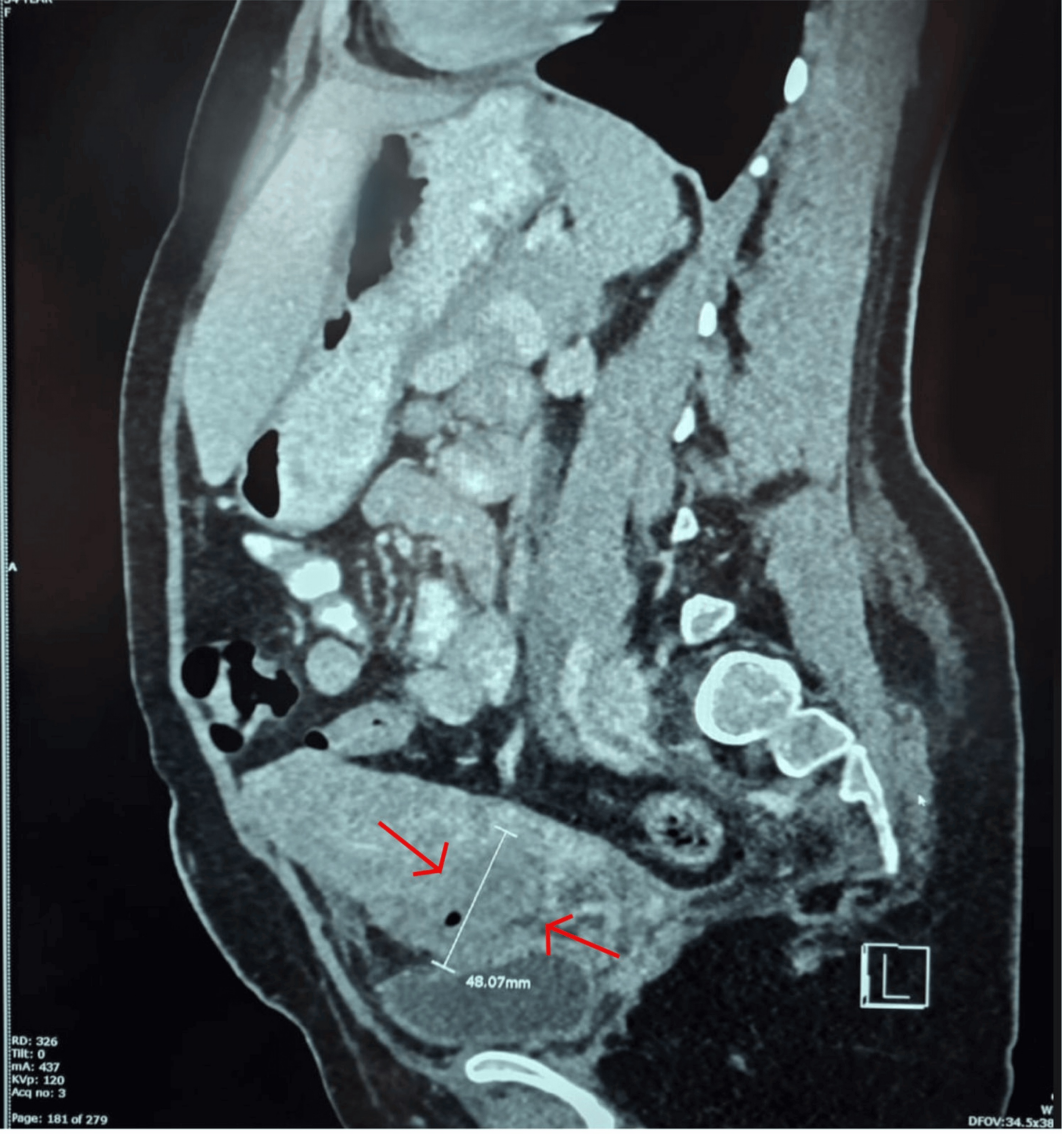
CT abdomen and pelvis showing a haematoma with air locules, extending from the lower endometrial cavity through the caesarean scar to the space between the uterus and urinary bladder.
